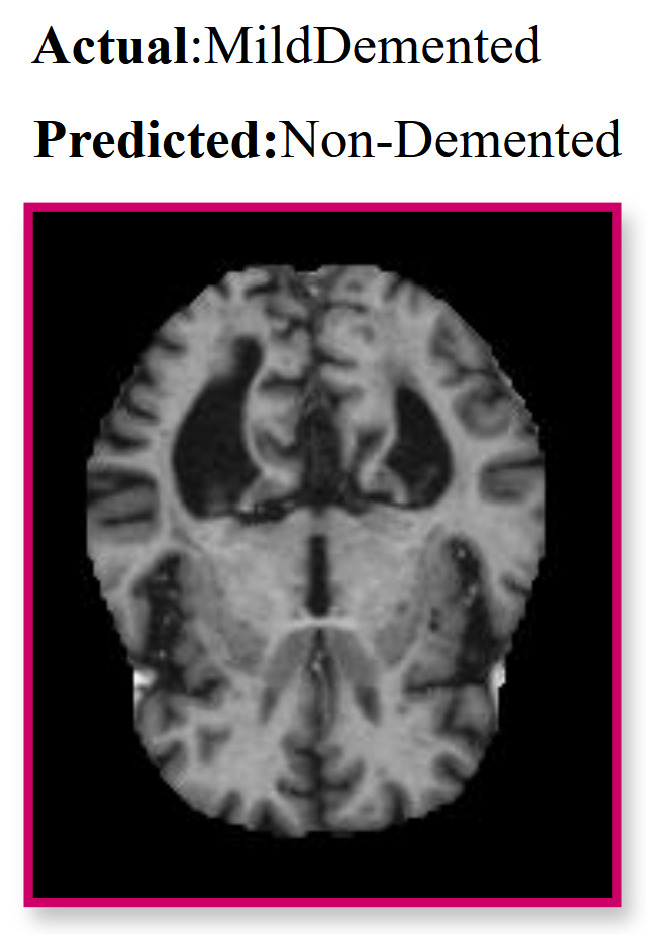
T1-weighted MRI with red border showing a misclassification: actual label Mild Demented, predicted as Non-Demented.
